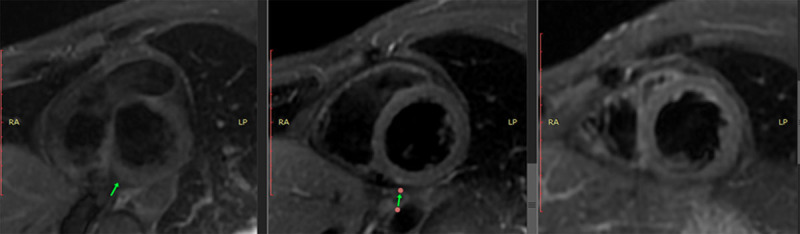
Cardiac MRI with triple IR sequencing showing hyperintense signal, compatible with myocardial edema.
